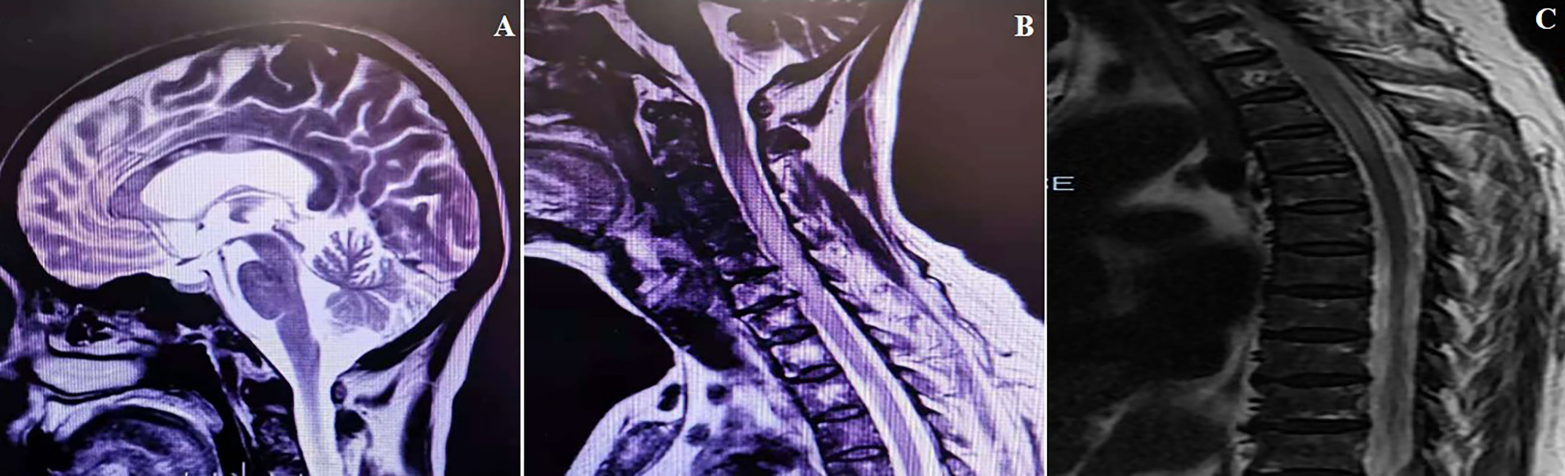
MRI scans obtained at the time of the first attack. (A) Cerebral MRI (sagittal T2 image) exhibiting disseminated lesions in corpus callosum. (B, C) Spinal MRI (sagittal T2 image) exhibiting longitudinally extensive lesion from C3 to T5.
